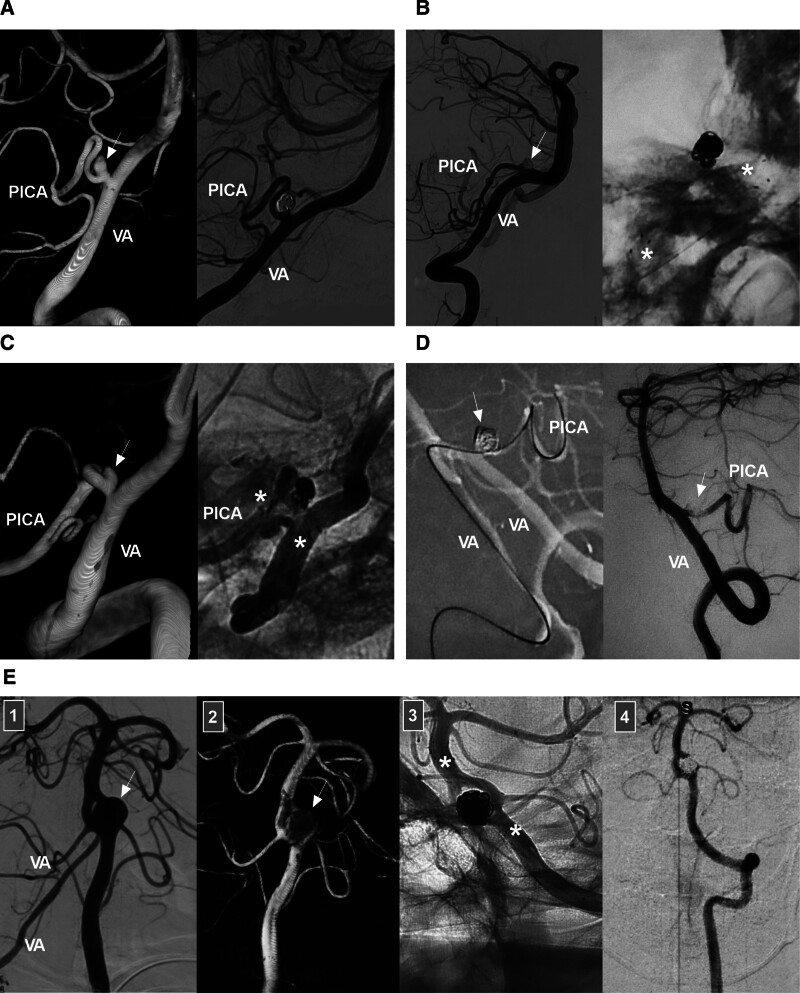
Endovascular treatment of aneurysms at the VA-PICA junction. (A) Coiling alone: left panel: DSA image showing an aneurysm (arrow) at the VA-PICA junction; right panel: follow-up DSA image showing complete occlusion of the aneurysm. (B) Stent-assisted coiling: left panel: DSA image showing a coiled saccular aneurysm (arrow) at the VA-PICA junction; right panel: X-ray image showing a stent (asterisks) deployed in the VA to cover the origin of the PICA. (C) Coiling assisted by antegrade stenting from the VA to the PICA: DSA image showing an aneurysm (arrow) at the VA-PICA junction; right panel: Unsubtracted DSA image showing coiling of the aneurysm via stenting (asterisks) from the ipsilateral VA to the PICA. (D) Coiling assisted by retrograde stenting from the contralateral VA to the PICA: Roadmap image showing a recurrently coiled saccular aneurysm (arrow) at the VA-PICA junction; the PICA was catheterized from the contralateral VA to await delivery of the stent; right panel: DSA image showing the coiled aneurysm (arrow). (E) panels 1 and 2: DSA and its reconstructive images showing a saccular aneurysm (arrows) in a VA fenestration; panel 3: DSA image showing coiling of the aneurysm with stenting assistance (asterisks); panel 4: follow-up DSA image showing complete occlusion of the aneurysm. DSA = digital subtraction angiography, PICA = posterior inferior cerebellar artery, VA = vertebral artery.
